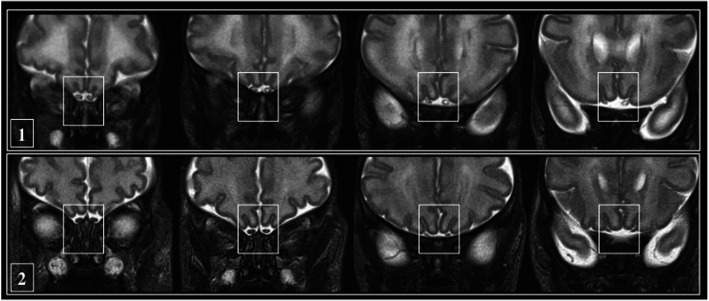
MRI coronal T2 weighted images: the OBs (squared white box) in the patient with CMV infection (row 1) showed abnormal T2 hyperintensity in the central portion in comparison with the physiological appearance (row 2) of OB of a newborn without CMV infection studied at the same corrected GA. CMV: Citomegalovirus, GA: gestational age; OB: olfactory bulb
